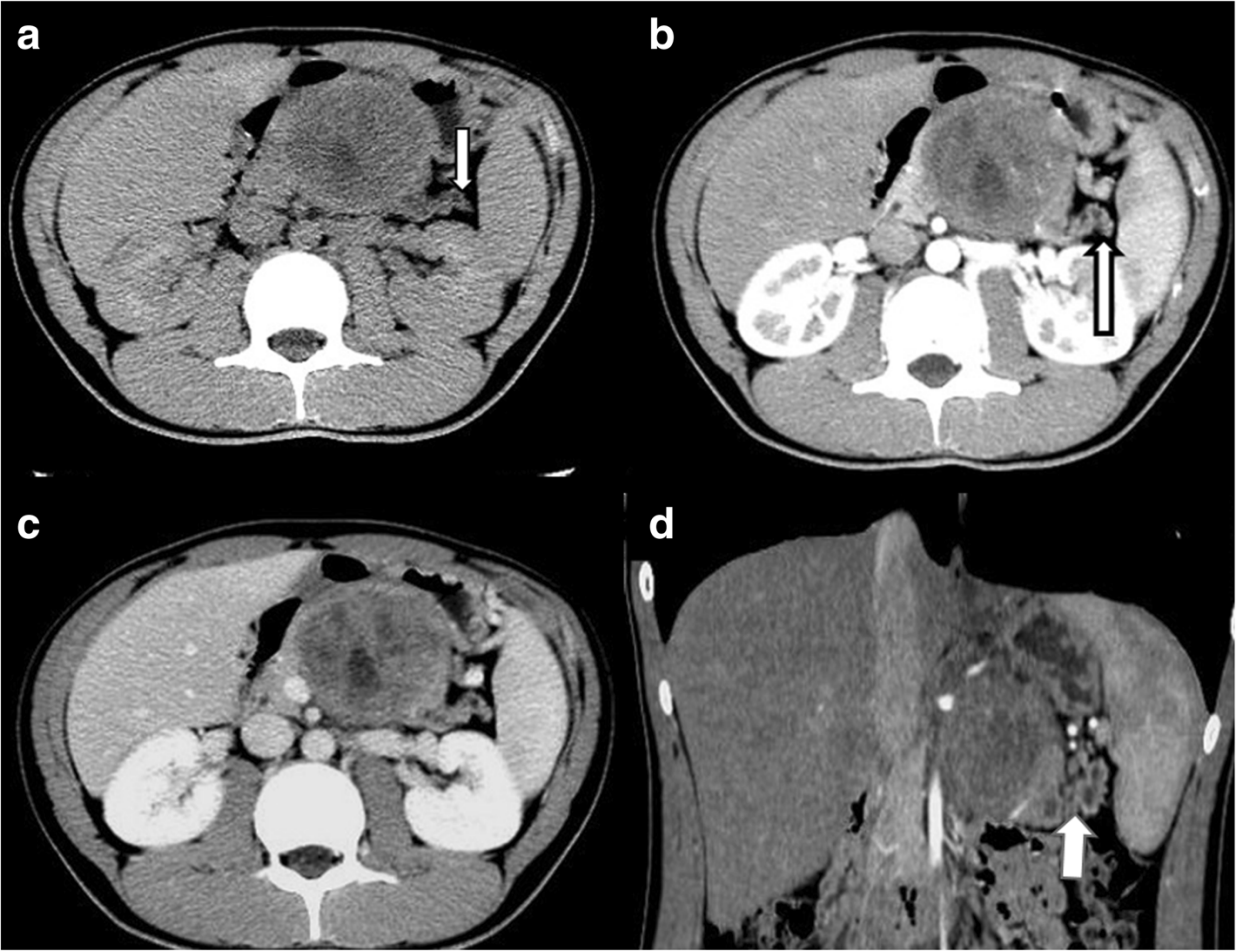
Abdominal CT scans of symptomatic SPT in a 21-year-old woman. a Unenhanced CT scan showing a large heterogeneous mass in the body of the pancreas, dilatation of the pancreatic duct and parenchymal atrophy (arrow) distal to the mass lesion in the body of the pancreas. b Scan obtained during the pancreatic phase. c Scan obtained during the hepatic venous phase showing mostly solid and small cystic areas at the center and a cystic mass without significant contrast enhancement. d Coronal pancreatic phase CT image showing dilatation of the pancreatic duct and parenchymal atrophy (arrow) distal to the mass lesion in the body of the pancreas
